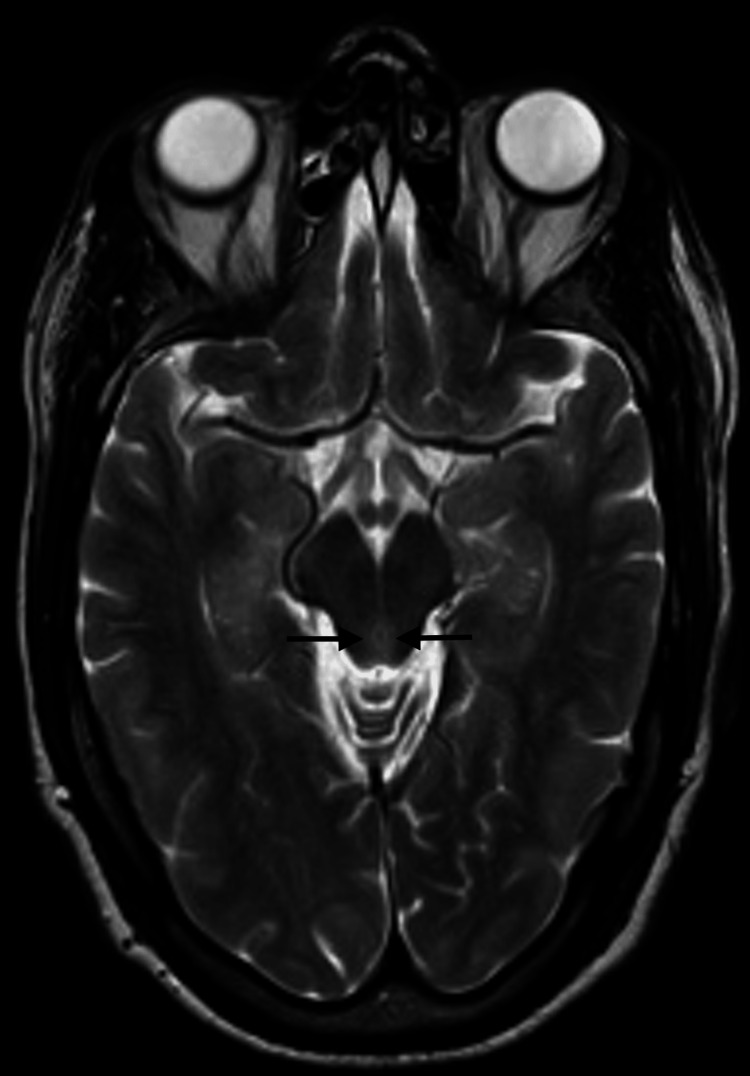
Contrast-enhanced MRI: Follow-up study. Axial T2 periaqueductal follow-up. Slight improvement in bilateral and symmetrical hyperintensity around the third ventricle in the periaqueductal gray matter is observed on T2-weighted images (arrows).
